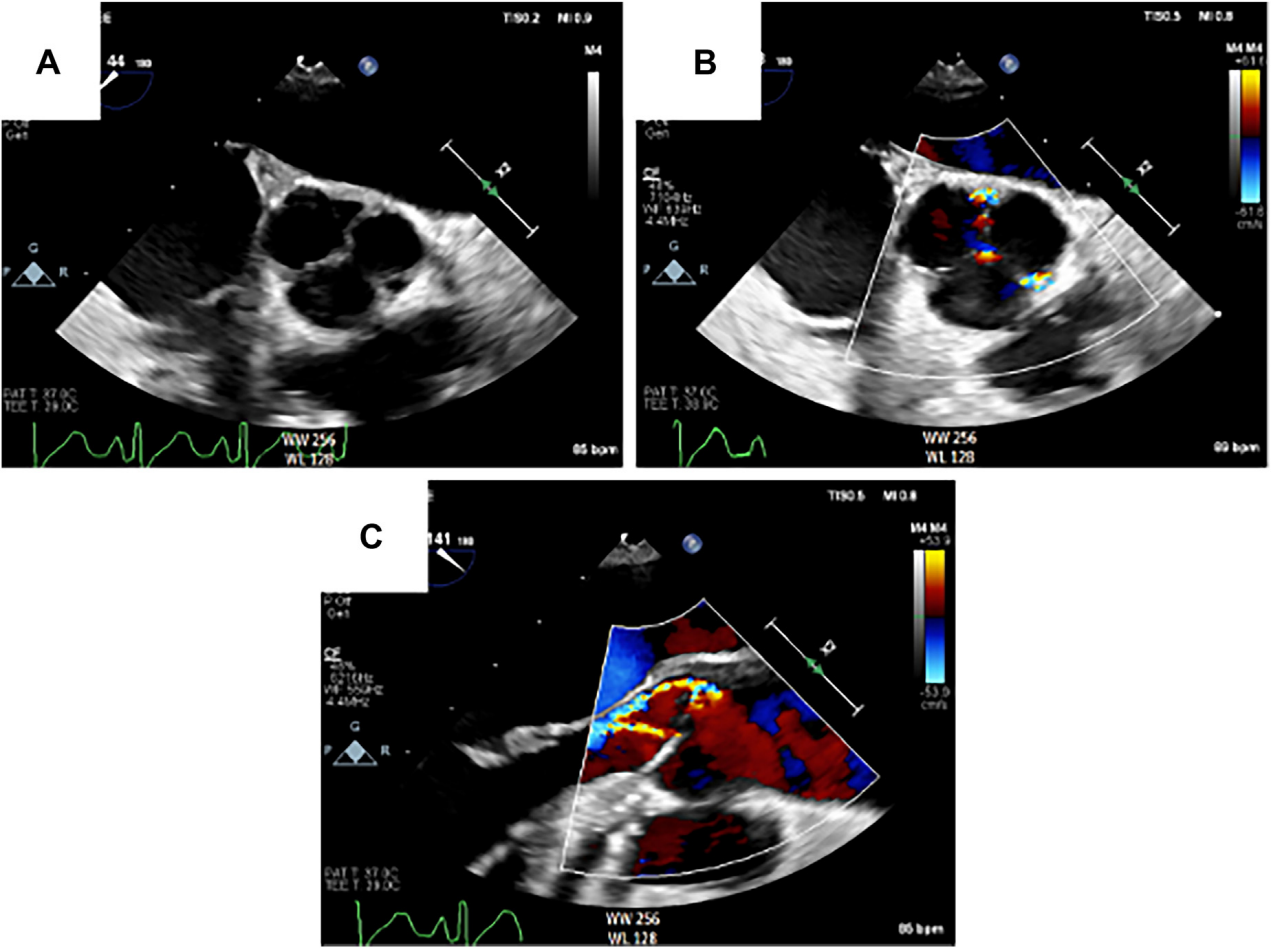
Intraoperative transesophageal echocardiography of the syphilitic aortic valve. (A) Midesophageal short-axis view of the aortic valve demonstrates thickening and contracture of aortic cusps. (B) Midesophageal short-axis view of the aortic valve with color Doppler shows central aortic regurgitation and aortic regurgitation at the left-non and left-right commissures. (C) Midesophageal long-axis view of the aortic valve with color Doppler.
